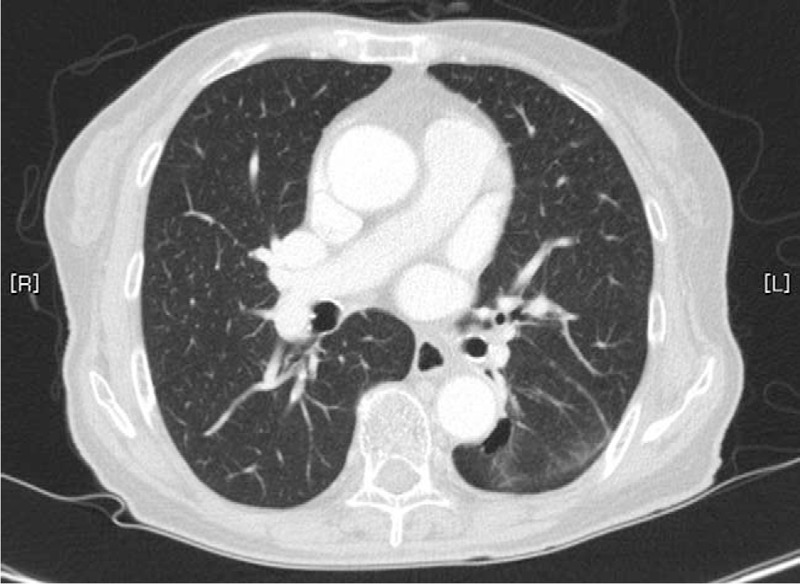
A follow-up chest computed tomography scan obtained on September 17, 2014, revealed a further decrease in the extent of the lymphangitic metastasis and pleural effusion. Thin-wall cavitation with a ground glass opacity is shown.
